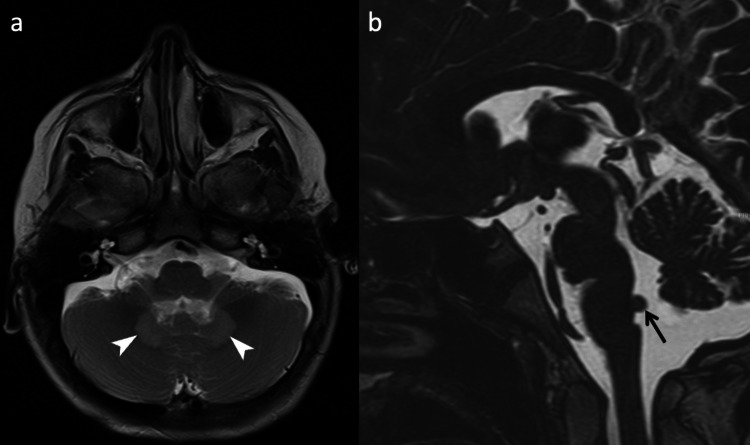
Neuroimaging findings in fibrodysplasia ossificans progressiva (FOP). a Axial T2-weighted magnetic resonance imaging (MRI) of a 19-month-old female (Patient 6) showing dentate nucleus hyperintensity (arrowheads). b Sagittal T2-weighted MR image of a 9-year-old male (Patient 8) demonstrating pontine dysmorphism with fusion of the facial colliculi and dorsal surface bulging into the fourth ventricle (arrows)
